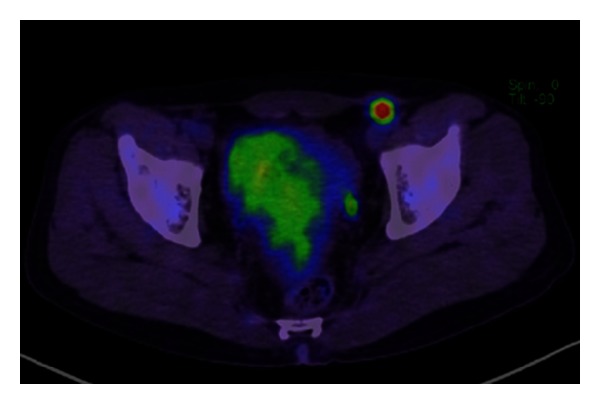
FDG-PET revealed concentration of FDG in the left inguinal area. The size of the mass was 1.1 cm.
